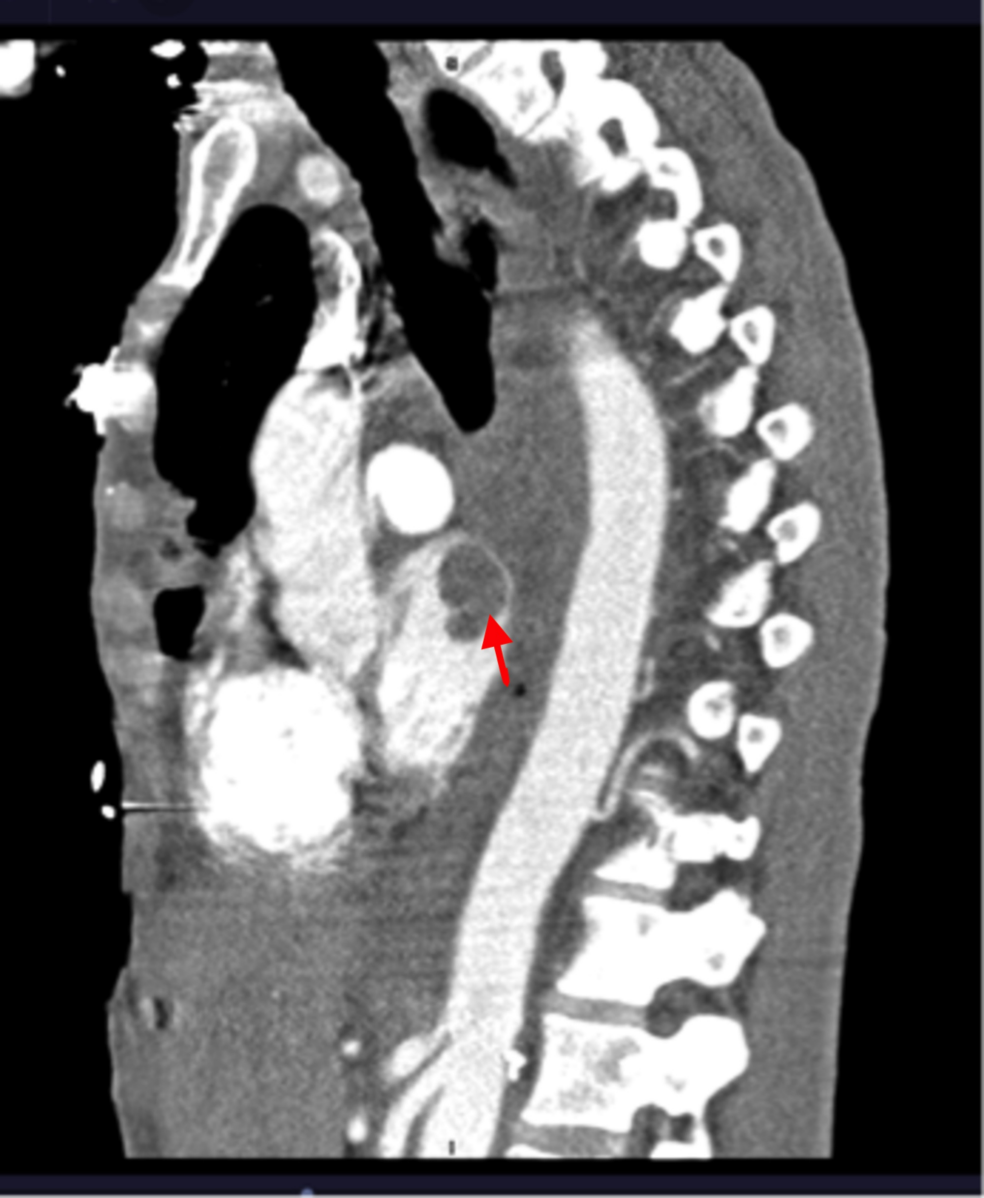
CT angiography of the chest showing tumor invasion to the left atrium
